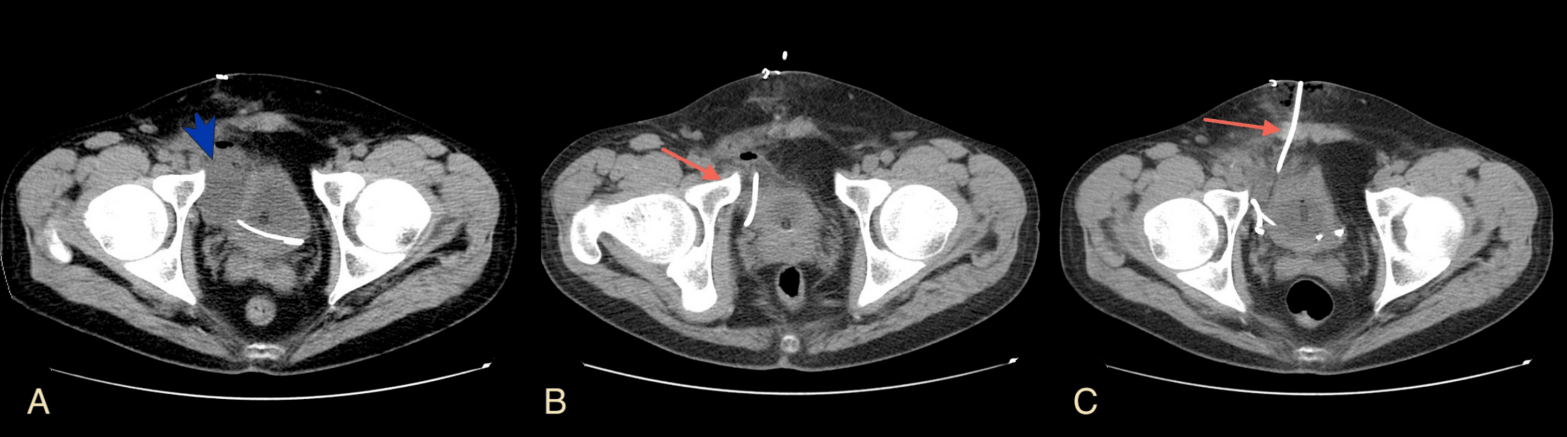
MDCT-guided placement of a drainage catheter in a urinoma one month after kidney transplantation (A) An axial multidetector computed tomography (MDCT) image without IV or retrograde contrast medium suggests a urinoma due to the low-density, round collection in contact with the bladder at the site of ureterovesical anastomosis (blue arrowhead). The bladder cavity contains the distal part of the ureteric stent and the Foley catheter cuff; (B, C) Axial MDCT images after the drainage catheter placement (red arrows). The urinoma was again confirmed by laboratory examination of the drained fluid. Image source: Department of Radiology, Ippokrateio General Hospital of Thessaloniki. Permission obtained for reproduction.
